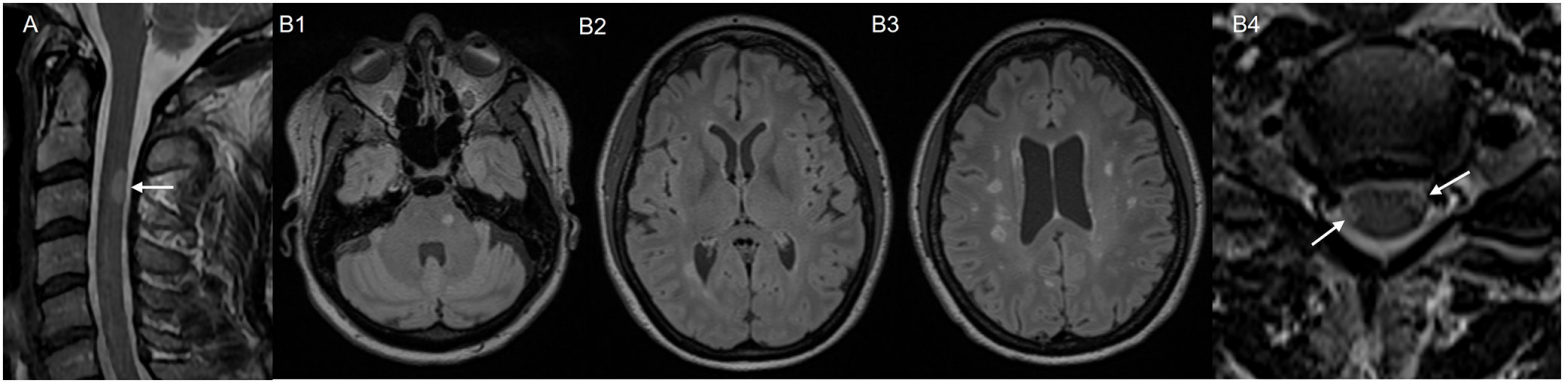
MRI images of two representative Mayo clinic cases. Case 1 with post-TNFI myelitis: (A) MRI cervical spine, sagittal T2 sequence, showing T2 hyperintense lesion in the posterior spinal cord at C3. Case 2 with post ICI-myelitis: (B1–B3) MRI brain, axial T2 fluid-attenuated inversion recovery (FLAIR) sequence, obtained prior to immune checkpoint inhibitor treatment showing multiple white matter T2 hyperintensities suggestive of demyelination. (B4) MRI cervical spine, axial T2 sequence, obtained after immune checkpoint inhibitor treatment, showing two spinal cord lesions suggestive of demyelination (arrows).
